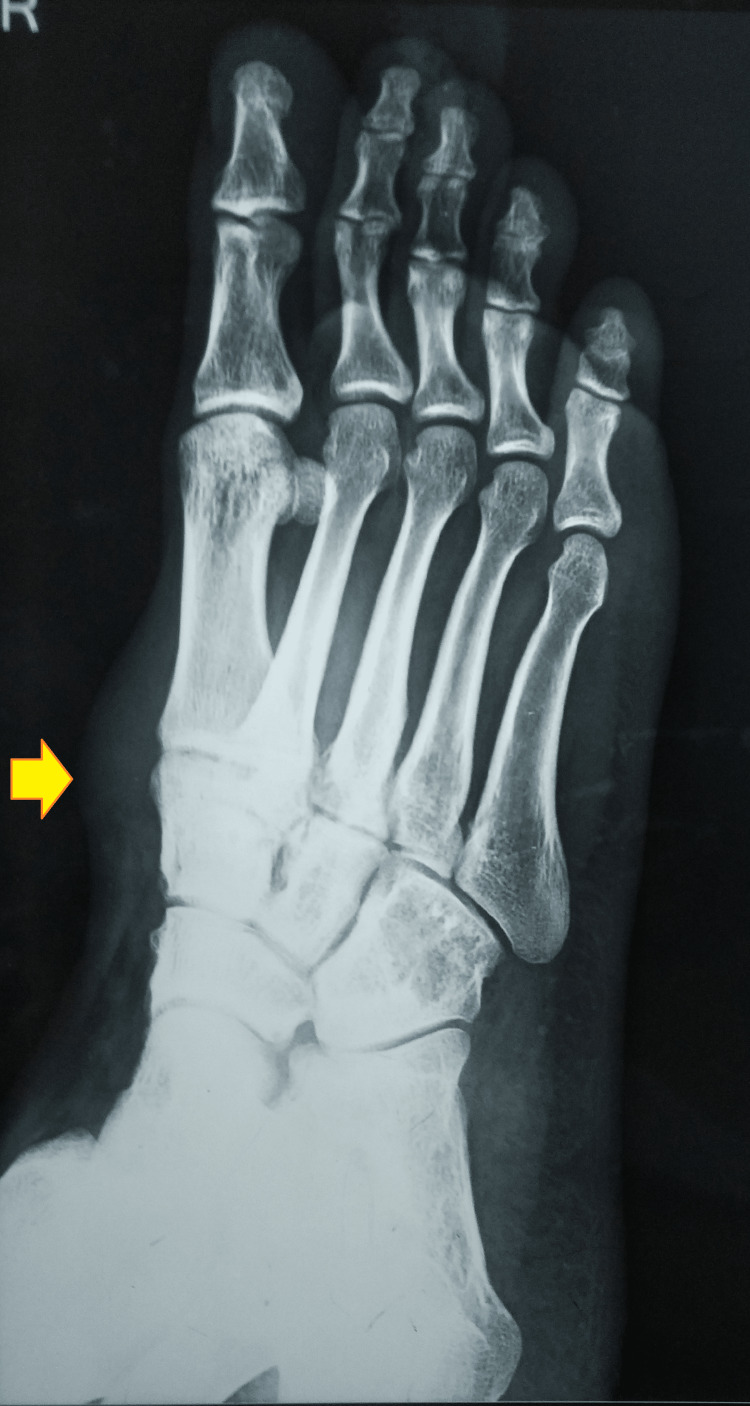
Oblique radiographs of the right foot showing the soft tissue swelling
